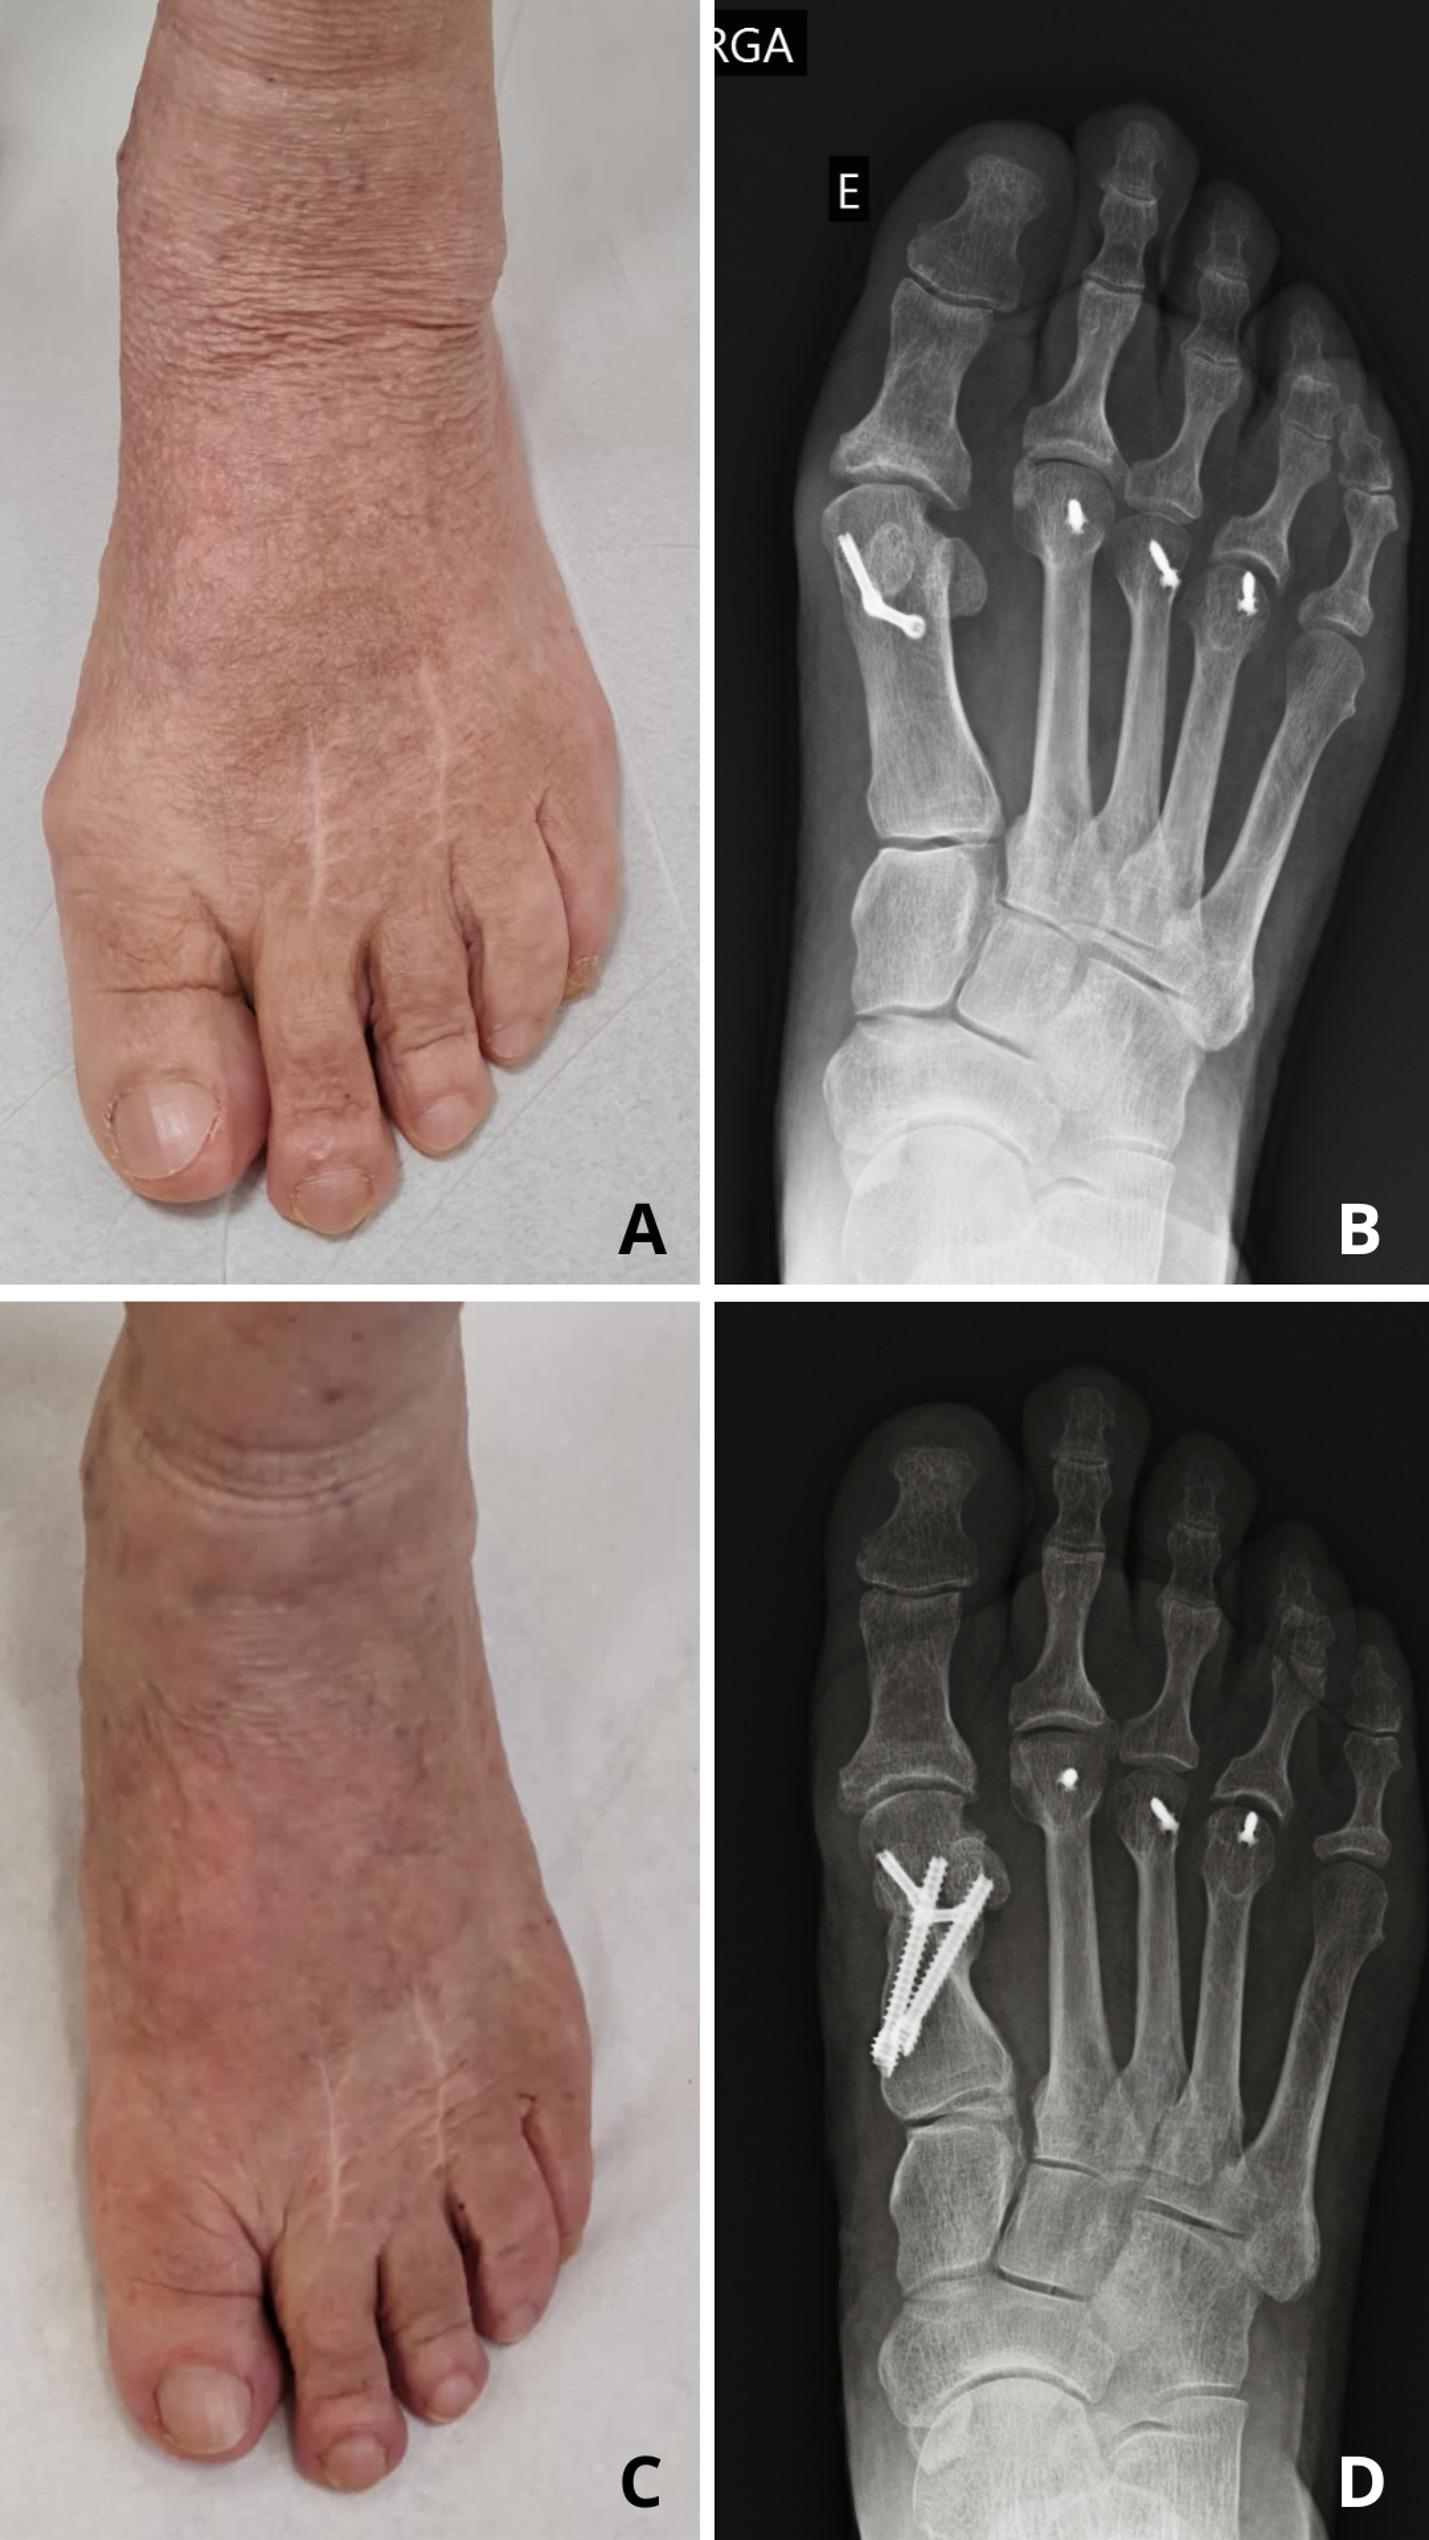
Clinical photographs and weightbearing anteroposterior (AP) radiographs. A Preoperative clinical image. B Preoperative AP radiograph. C Postoperative clinical image. D Postoperative AP radiograph after minimally invasive revision
